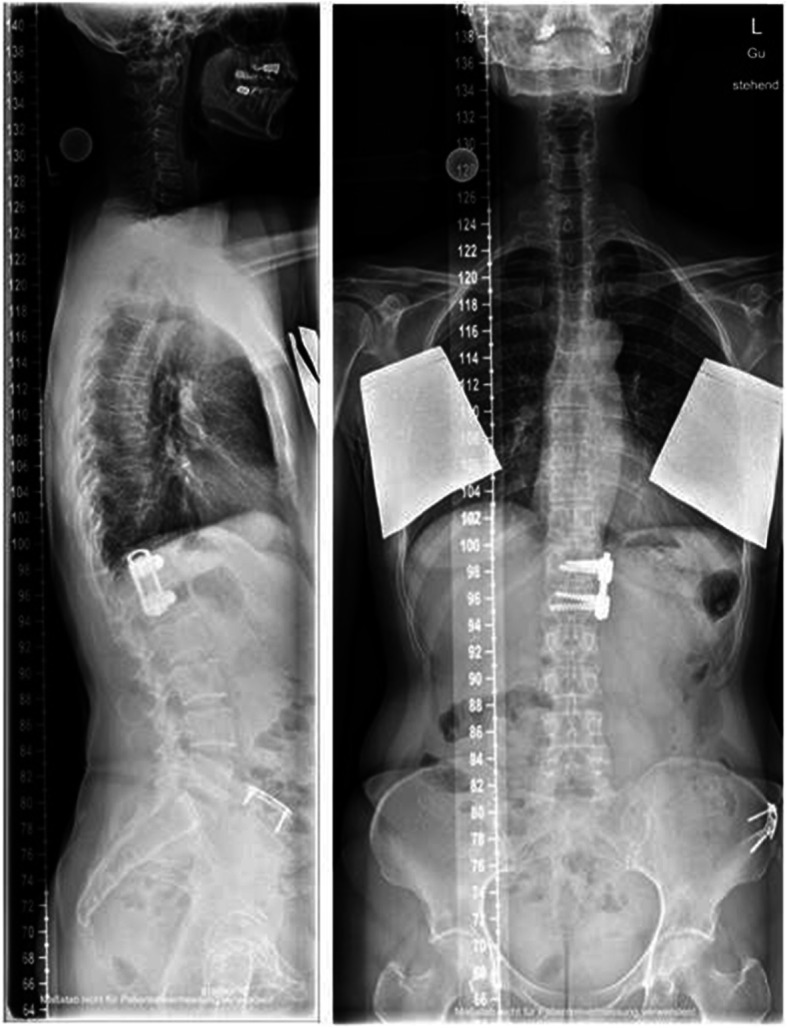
Full spine radiograph showing long-term radiological follow-up after monosegmental anterior spondylodesis with a tricortical iliac crest bone graft and MACS plate. Plate osteosynthesis of the iliac crest was performed to restore the shape of the iliac crest
